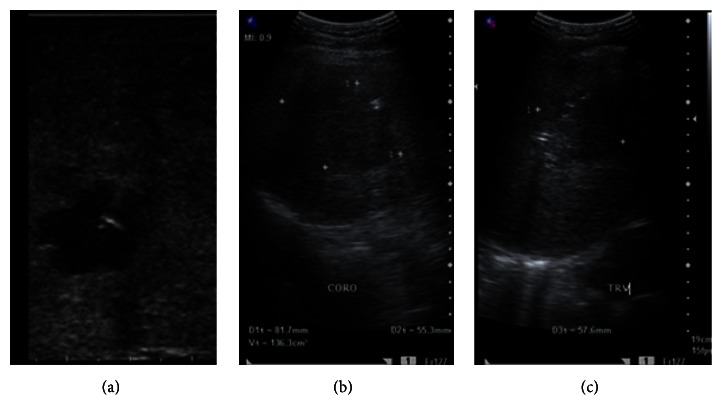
(a) Laparoscopic ultrasound showing the placement of a pigtail catheter to the liver abscess located at segment 8. ((b) and (c)) Third day's postoperative ultrasound showing decrease in the amount of abscess from 162 cc to 136 cc.
